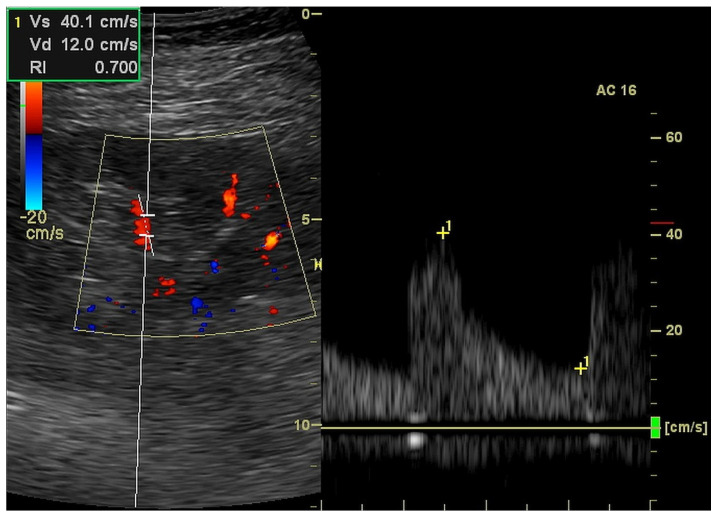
Ultrasound measurement of resistive index in the triplex (2D, color Doppler and pulse wave Doppler) mode.
